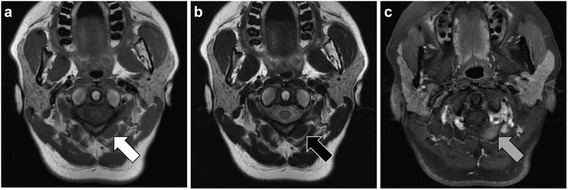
Axial MRI of a soft tissue mass (14.6 × 7.7 × 7 mm) adjacent to the C1 lamina showed isointensity on a T1WI (*white arrow*) (a), low intensity on a T2WI (*black arrow*) (b), and enhanced gadolinium uptake (*gray arrow*) (c)
